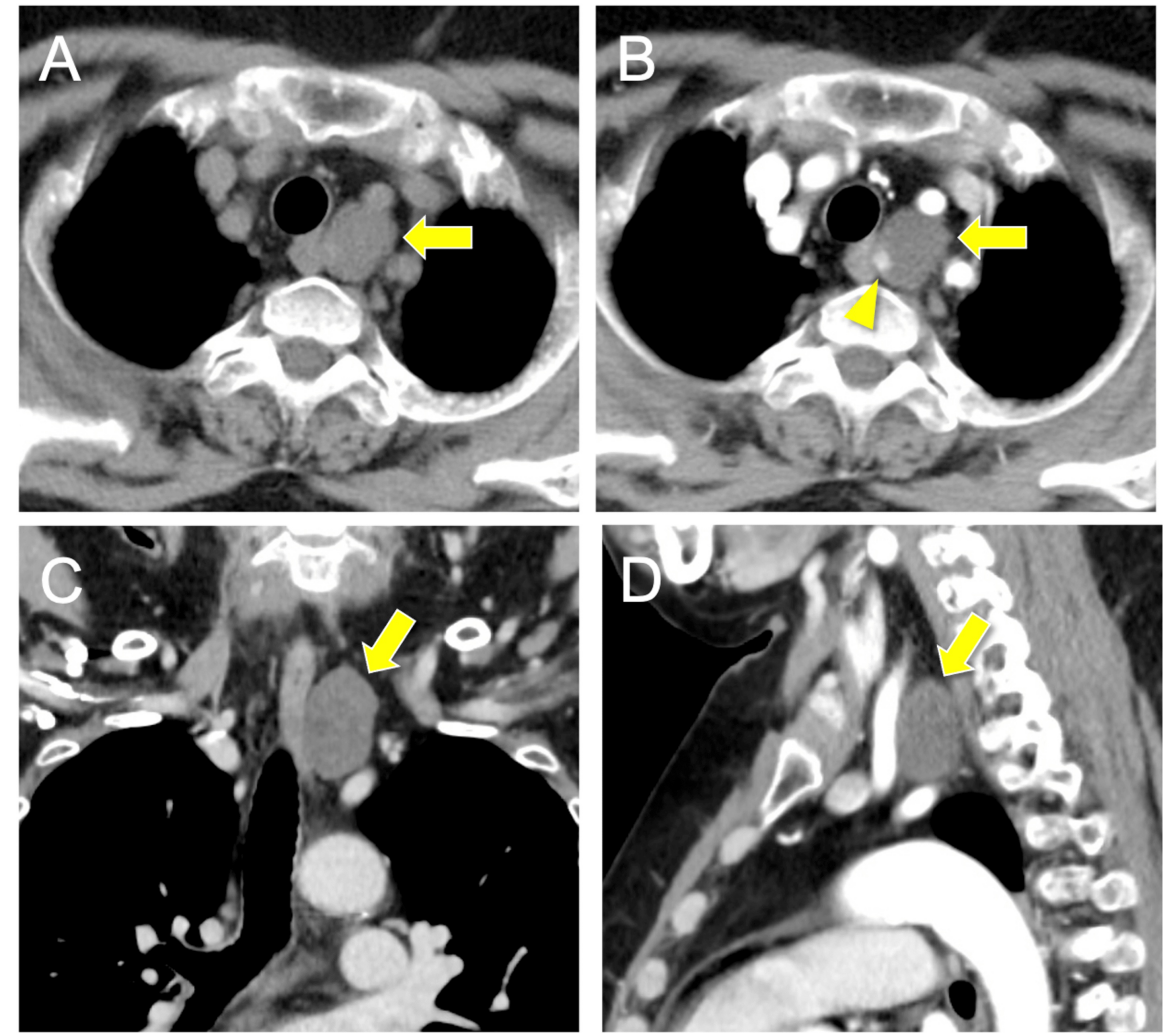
Preoperative CT images (A) A non-enhanced CT scan showed a mass in the superior mediastinum near the left side of the esophagus. (B) Contrast-enhanced CT showed that most of the mass was non-enhancing, but a small portion within the mass was contrast-enhancing (arrowhead). (C) Coronal section and (D) sagittal section of contrasted CT. The mass was located posterior to the common carotid artery and mediosuperior to the subclavian artery.
